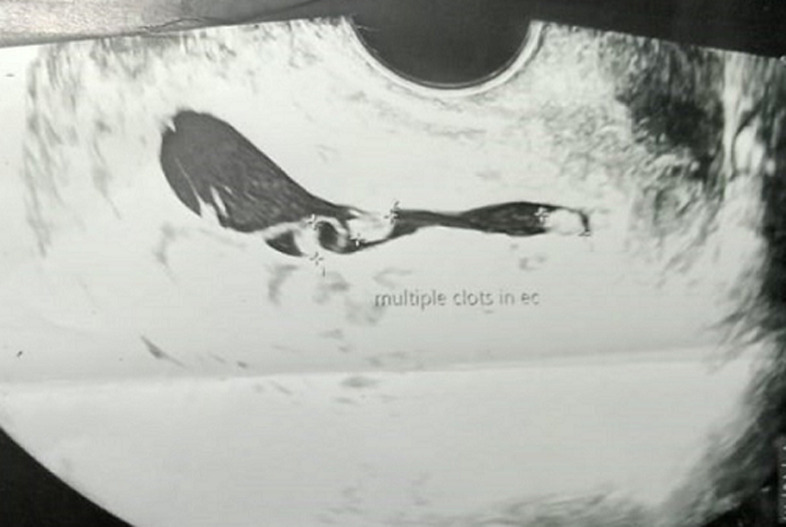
trans abdominal sonography demonstrating multiple clots in endometrial cavity
